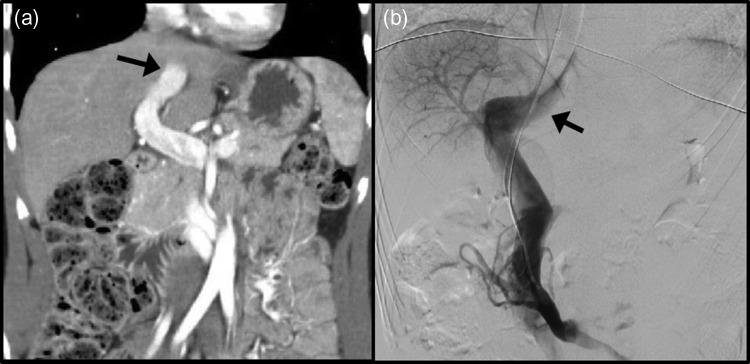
Radiological assessment of the patent *ductus venosus* (PDV) by (a) computed tomography and (b) digital subtraction angiography. Black arrow: PDV traversing towards the inferior vena cava.
